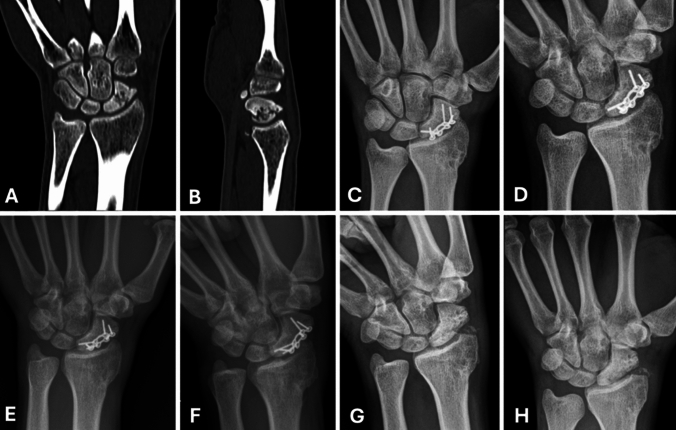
Radiological sequence of a patient who suffered acute trauma, with a scaphoid waist fracture treated with volar plate fixation. A. Coronal view of a preoperative computed tomography (CT) scan of the right wrist. B. Sagittal CT view showing a displaced scaphoid waist fracture. C. Posteroanterior (PA) radiograph at 3 months postoperatively. D. PA view with ulnar deviation at 3 months, demonstrating radiographic signs of bone consolidation. E. PA view at 6 months of follow-up. F. PA view with ulnar deviation at 6 months, confirming continued radiographic consolidation. G. PA view with ulnar deviation at 12 months of follow-up. H. PA view at 12 months, after Implant removal (performed at 11 months), with maintained bone union
